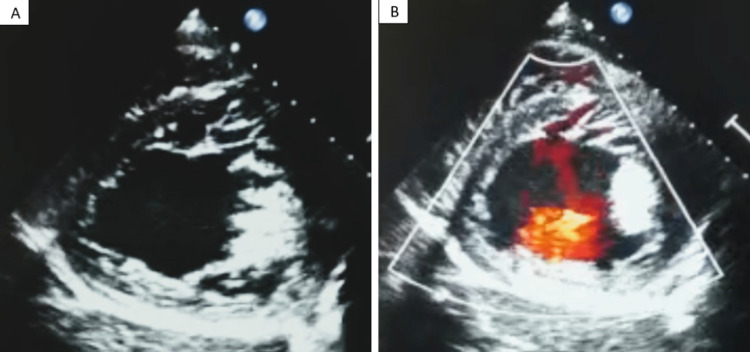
(A) Short-axis view showing LV hypertrabeculation in end systole. (B) Deep color Doppler passage within recesses. LV: left ventricular.
